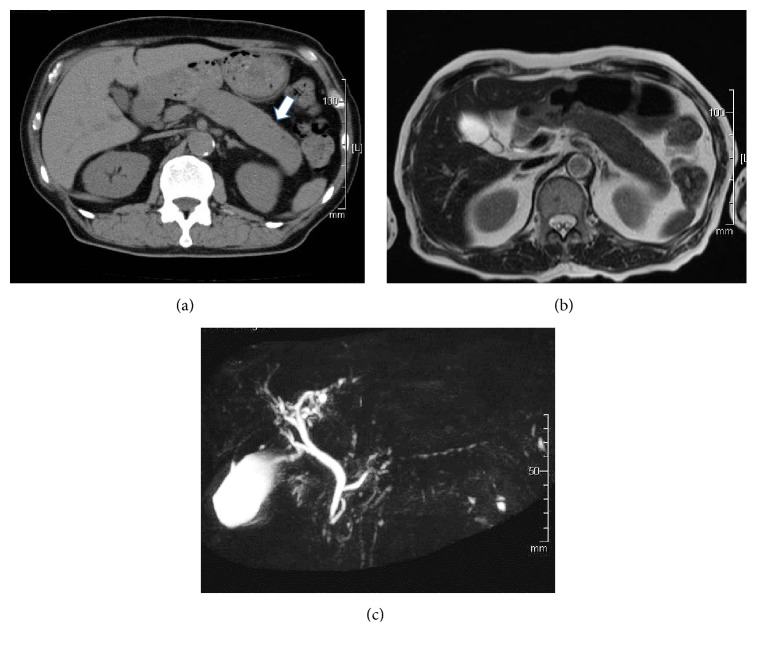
(a) An abdominal computed tomography scan performed on the patient's first visit shows diffuse swelling extending from the pancreatic body to tail. The arrow indicates the affected portion of the pancreas. (b) Magnetic resonance image (T2 weighted image) of the abdomen prior to prednisolone therapy reveals diffuse swelling extending from the pancreatic body to tail. (c) MRCP prior to prednisolone therapy shows narrowing of the main pancreatic duct extending from the pancreatic body to tail.
